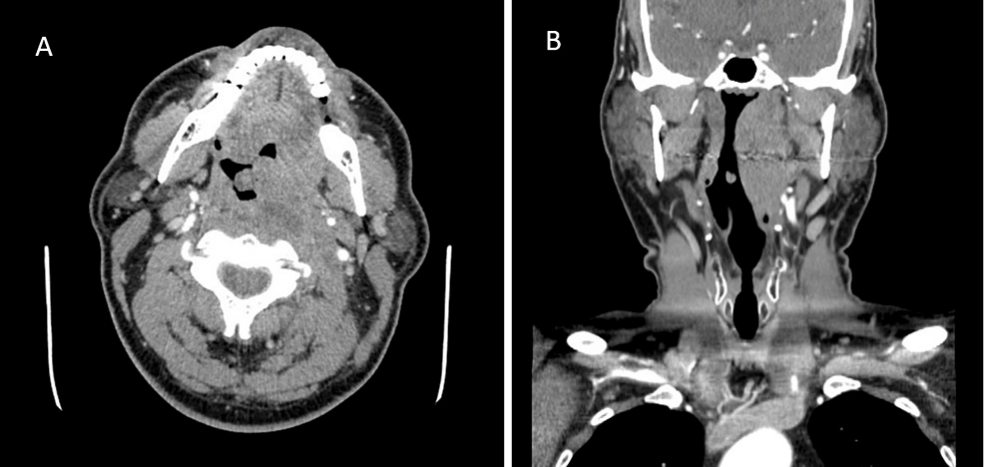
Initial CT head and neck performed on admission showing concern for airway-compromising mass, abscess versus malignancy
